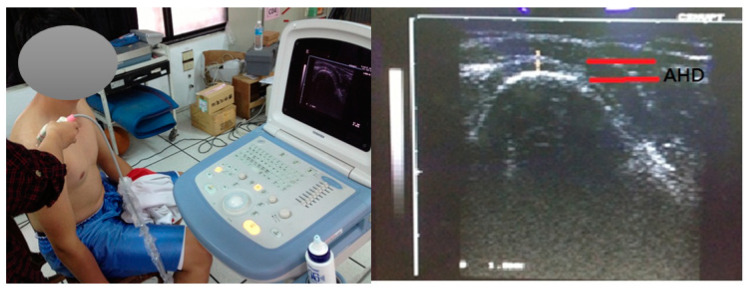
Sonographic images showing a measurement of the sub-acromial space. AHD: acromion to humeral head distance.
